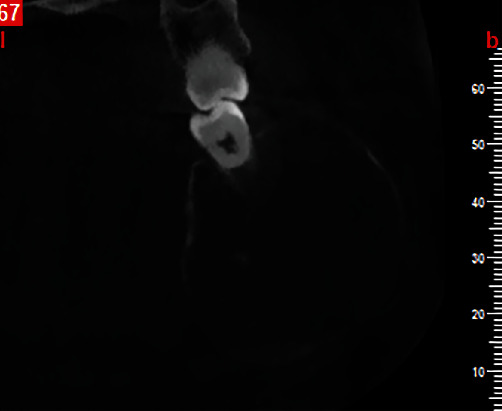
Cross-sectional CBCT image upon presentation.
